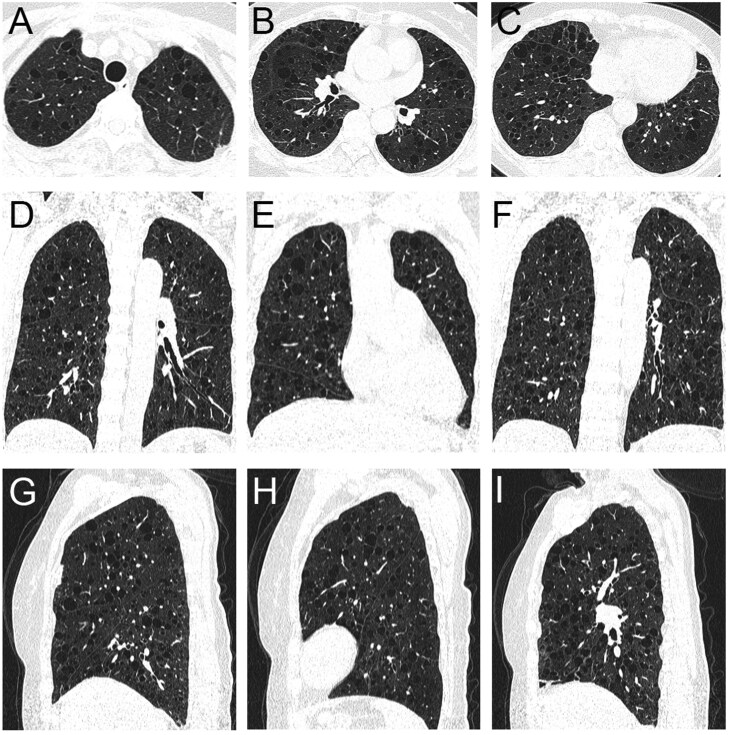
LAM in a 67-year-old woman. Axial (A-C), coronal (D-F), and sagittal (G-H) CT images demonstrate numerous smooth, thin-walled, round, or oval cysts evenly distributed across both lungs, including the costophrenic angles. The cysts lack internal septa or traversing vessels, and the intervening lung parenchyma appears normal. Abbreviation: LAM = lymphangioleiomyomatosis.
